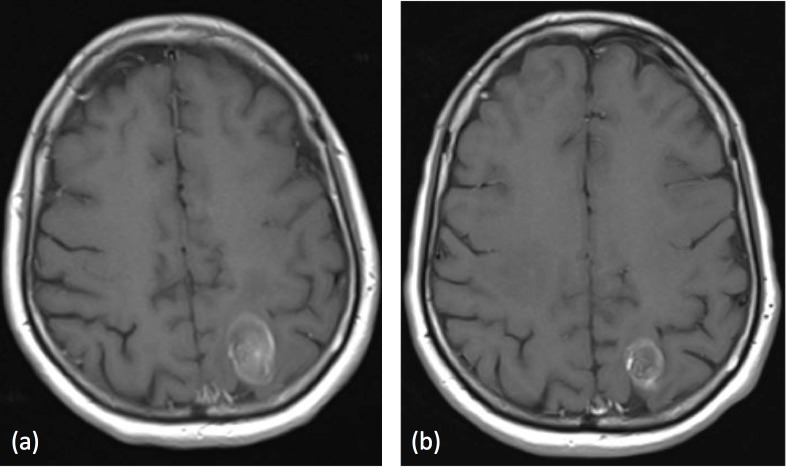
MRI head following LITT (a) Left parietal lesion measuring 28.3 x 18.5 x 20.3 mm six weeks post-LITT; (b) Left parietal lesion measuring 19.4 x 15.1 x 20.1 mm six months post-LITT MRI: magnetic resonance imaging; LITT: interstitial thermal therapy
